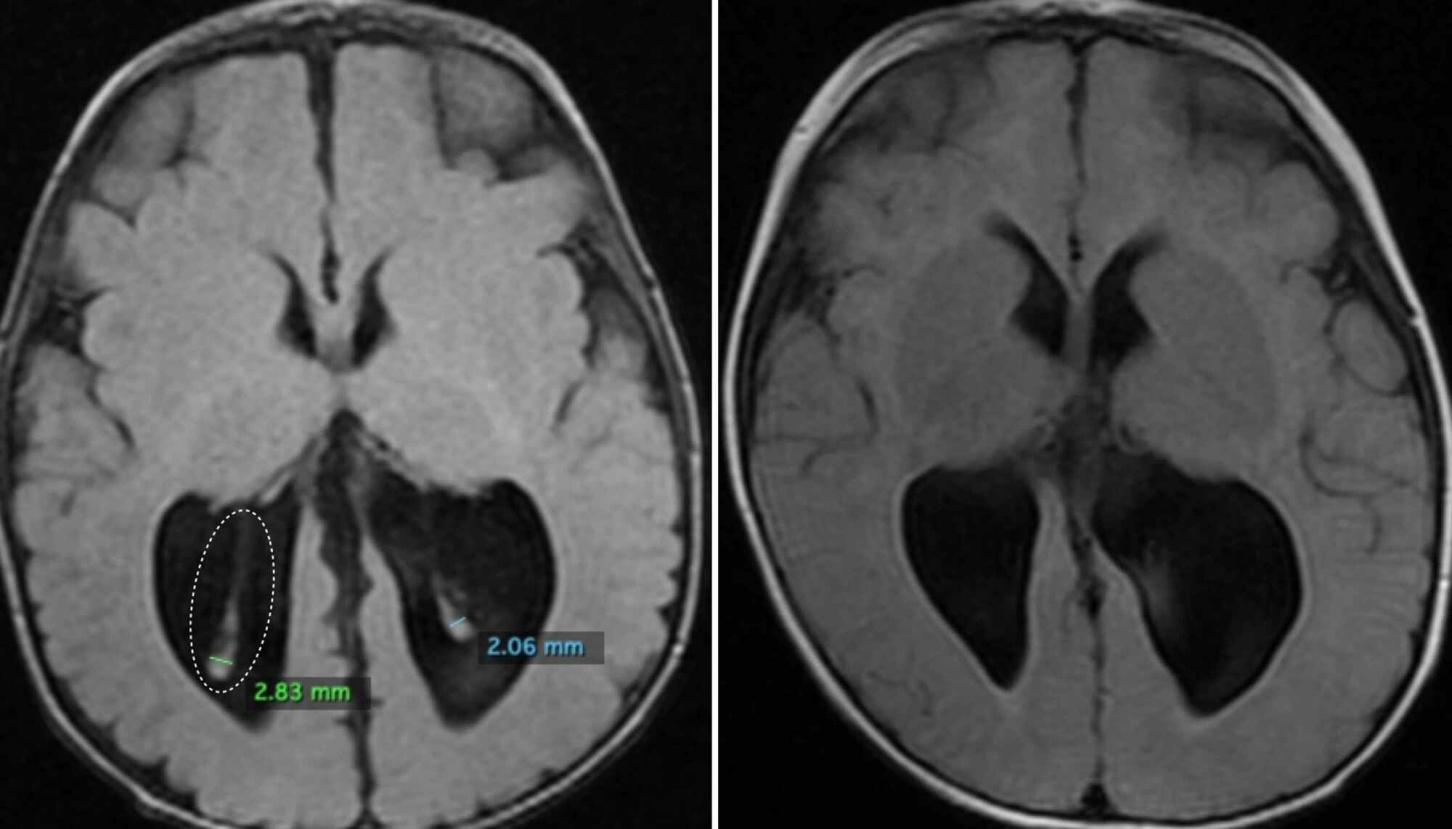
Outcome of selective choroid plexus resection. A: Axial T1-weighted magnetic resonance imaging before (left) and one year after endoscopic third ventriculostomy performed with choroid plexus cauterization and resection of the type III glomus of the choroid plexus (right). The patient was born with myelomeningocele, which was repaired in the first 24 hours after birth. Symptoms of hydrocephalus appeared when the patient was two months old, and the neuroendoscopic approach was indicated. The hydrocephalus symptoms resolved without a shunt, and imaging confirmed satisfactory control of cerebrospinal fluid.
